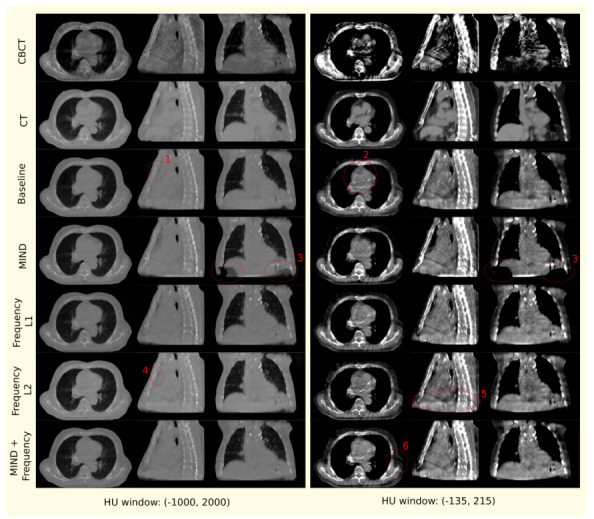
Mid−axial, sagittal, and coronal views for CBCT, CT, and generated images from models with different data-driven constraints for a patient chosen randomly from the test set. Qualitative observations discussed in the text are numbered and marked with red dotted ellipses.
